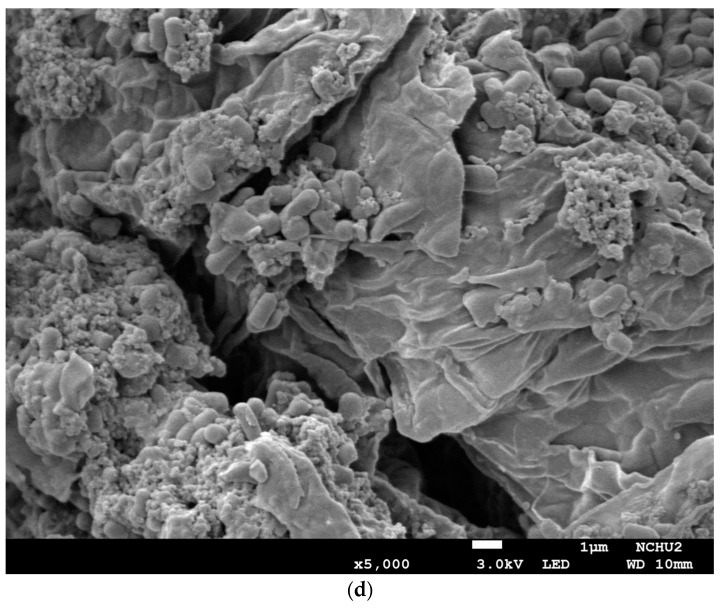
FE-SEM images of the solids separated from the medium in 12 h with *L. plantarum* BCRC 10357: (a) Oka-US; (b) 1%ChCl (no US); (c) 1%ChCl-US; (d) 1%ChCl-USP. Oka: the medium of MRS combined with 3% black soybean okara; Oka-US: the Oka medium with ultrasonic treatment (40 kHz/300 W) at 60 °C for 3 h before inoculation; 1%ChCl (no US): the Oka medium combined with 1% choline chloride (ChCl) without ultrasonic treatment; 1%ChCl-US: the Oka medium combined with 1% ChCl using ultrasonic treatment (40 kHz/300 W) at 60 °C for 3 h before inoculation; 1%ChCl-USP: the medium of 1%ChCl-US using *L. plantarum* BCRC 10357 that had been pretreated with an ultrasound probe (20 kHz/200 W) at 25 °C for 2 min.
